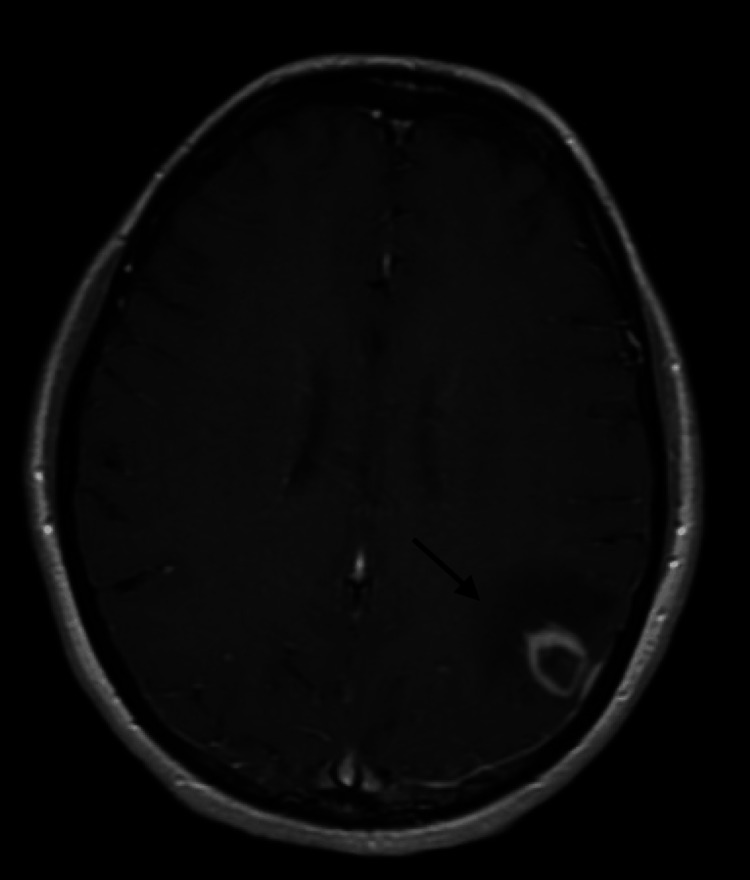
MRI of the brain showing heterogeneously enhancing and centrally non-enhancing lesion within the left parietal lobe. MRI: magnetic resonance imaging
